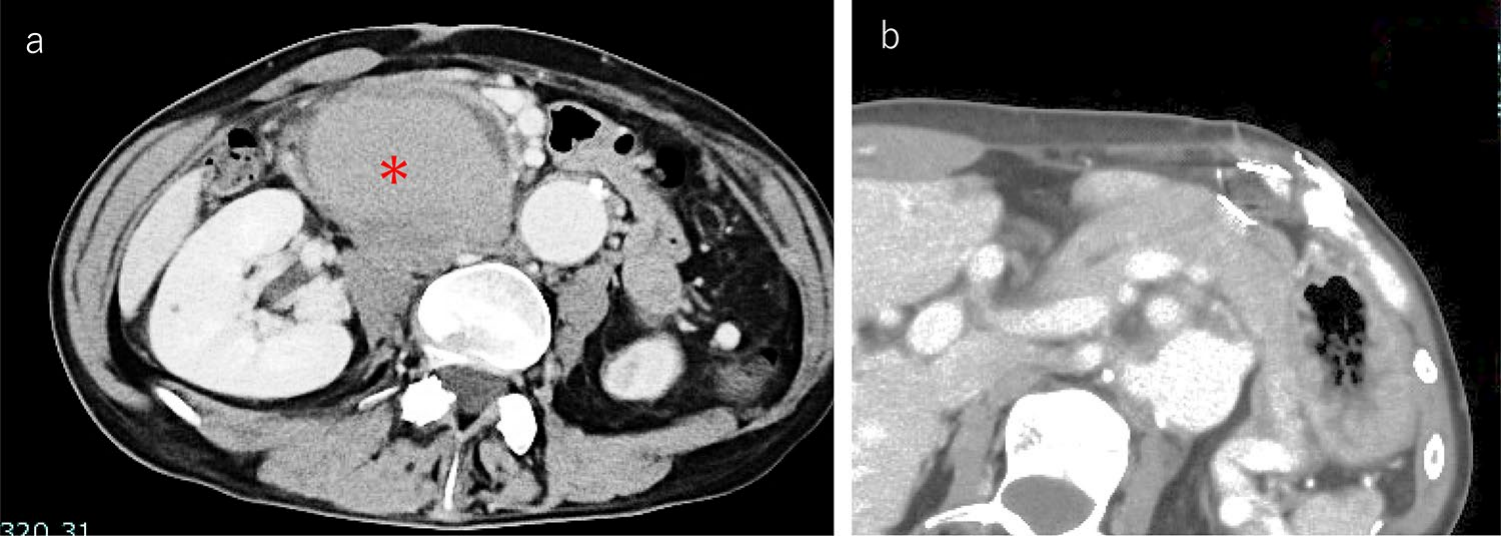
Computed tomography at the delayed phase showing a pseudoaneurysm compressing the pancreatic head and bile duct. a CT revealed a 70 mm pseudoaneurysm (*) arising from the posterior superior pancreaticoduodenal artery, which exhibited delayed contrast enhancement, indicating residual blood flow. And it compressed the pancreatic head. b CT showed slight dilation of the main pancreatic duct and pancreatic swelling
